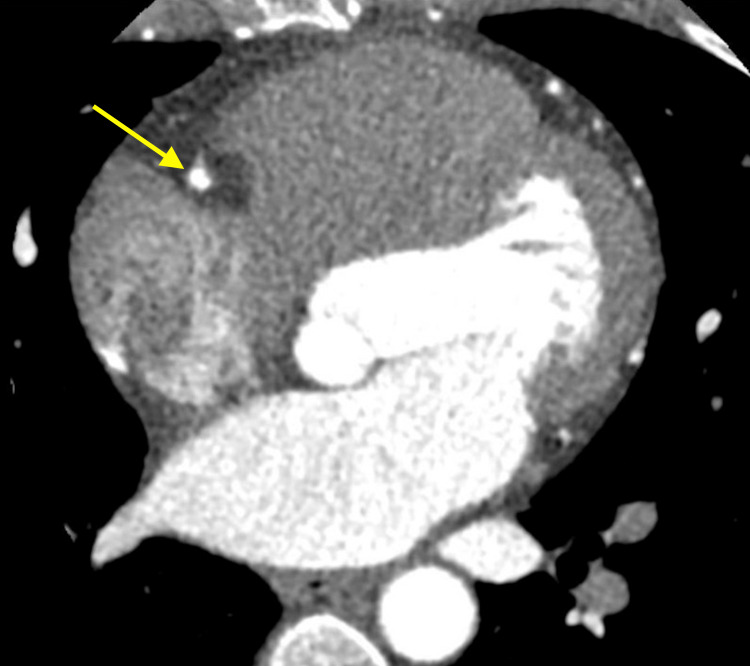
Repeat CCTA with mild RCA stenosis (yellow arrow), highlighting the spontaneous resolution of SCAD and prior severe RCA stenosis CCTA: coronary computed tomography angiography; RCA: right coronary artery; SCAD: spontaneous coronary artery dissection
